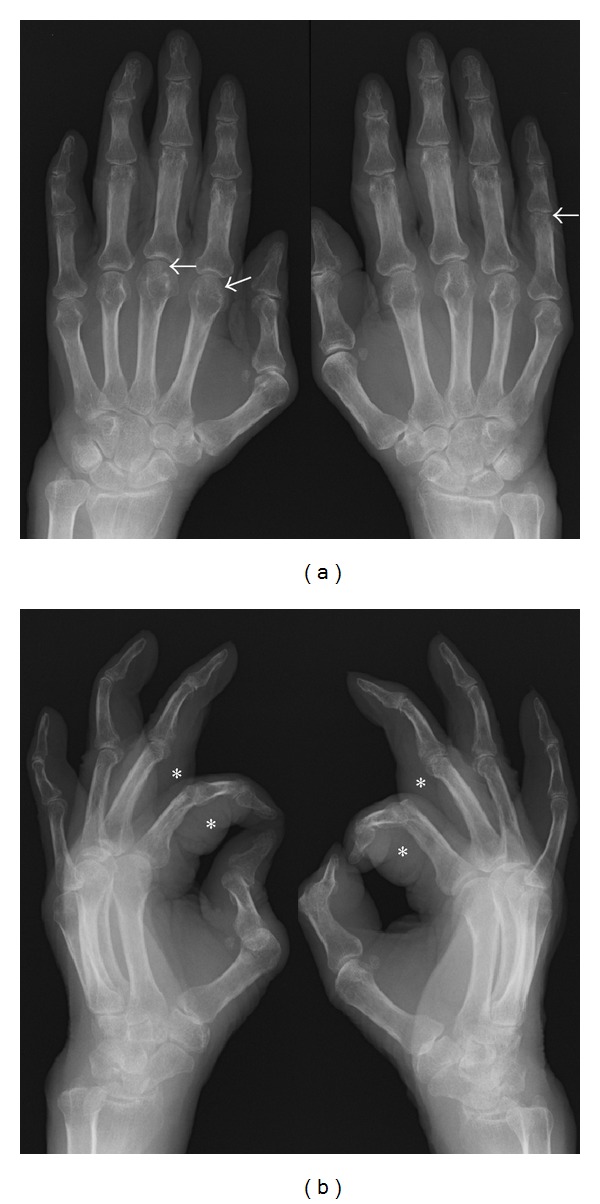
Plain radiographs of hands in Case 3. Plain radiographs of hands before treatment in the anterior-posterior view (a) and the oblique view (b). Arrows indicate small erosions in the heads of left 2nd and 3rd metacarpal bones and right proximal phalanx. Markedly thickened soft tissues are demonstrated (asterisks).
